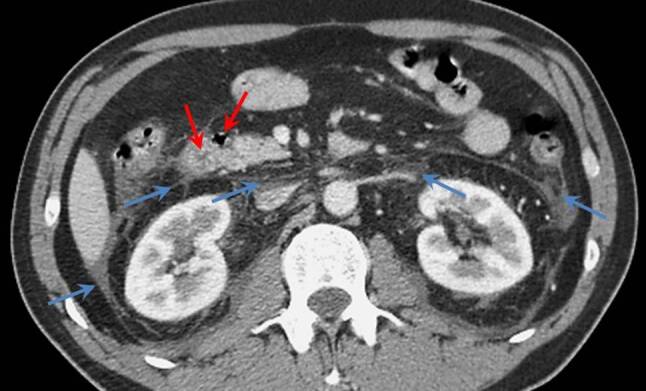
Contrast-enhanced abdominal CT scan in the axial plane demonstrating air-filled duodenal diverticulum with local mild duodenal wall thickening (*red arrows*) and fluid collections (*blue arrows*)
